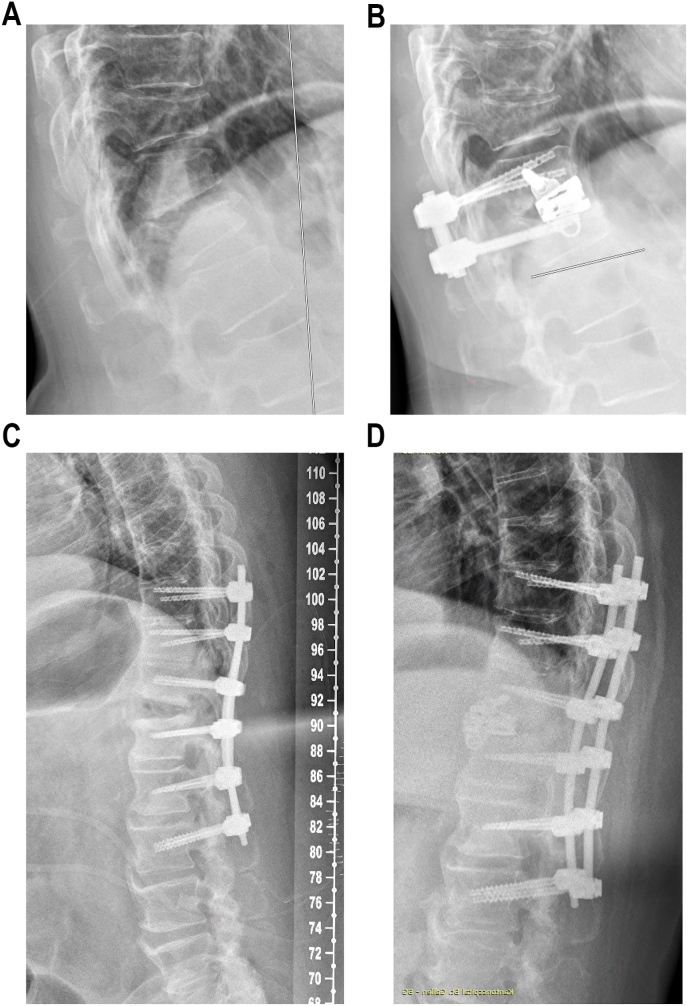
Typical case vignette of a 62-year-old female with history of prior, healed atraumatic T10 fracture (OF Type 2) and acute, kyphosing incomplete burst fracture (AO Spine Type A3) of Th12. A: Sagittal x-rays preoperative showing the segmental kyphosis at T11-T12 of 28°. B: Sagittal x-rays 3 months postoperative showing a reduction in segmental kyphosis of 10° after implantation of a 55 mm expandable lateral interbody cage (5–20° lordotic) with ALL release and posterior monosegmental fusion. Furthermore, a case vignette of a 59-year-old female with hyperextension fracture AO Spine Type B3 at T11-T12 in ankylosing spondylitis and prior instrumentation from T9-L2 is presented. C: In the immediate postoperative images we noticed a large residual gap with 20° lordosis, hindering fusion of the anterior column. D: After LLIF T11-T12 with a 55 mm expandable implant (5–20° lordotic) the gap was closed, helping to promote solid fusion.
